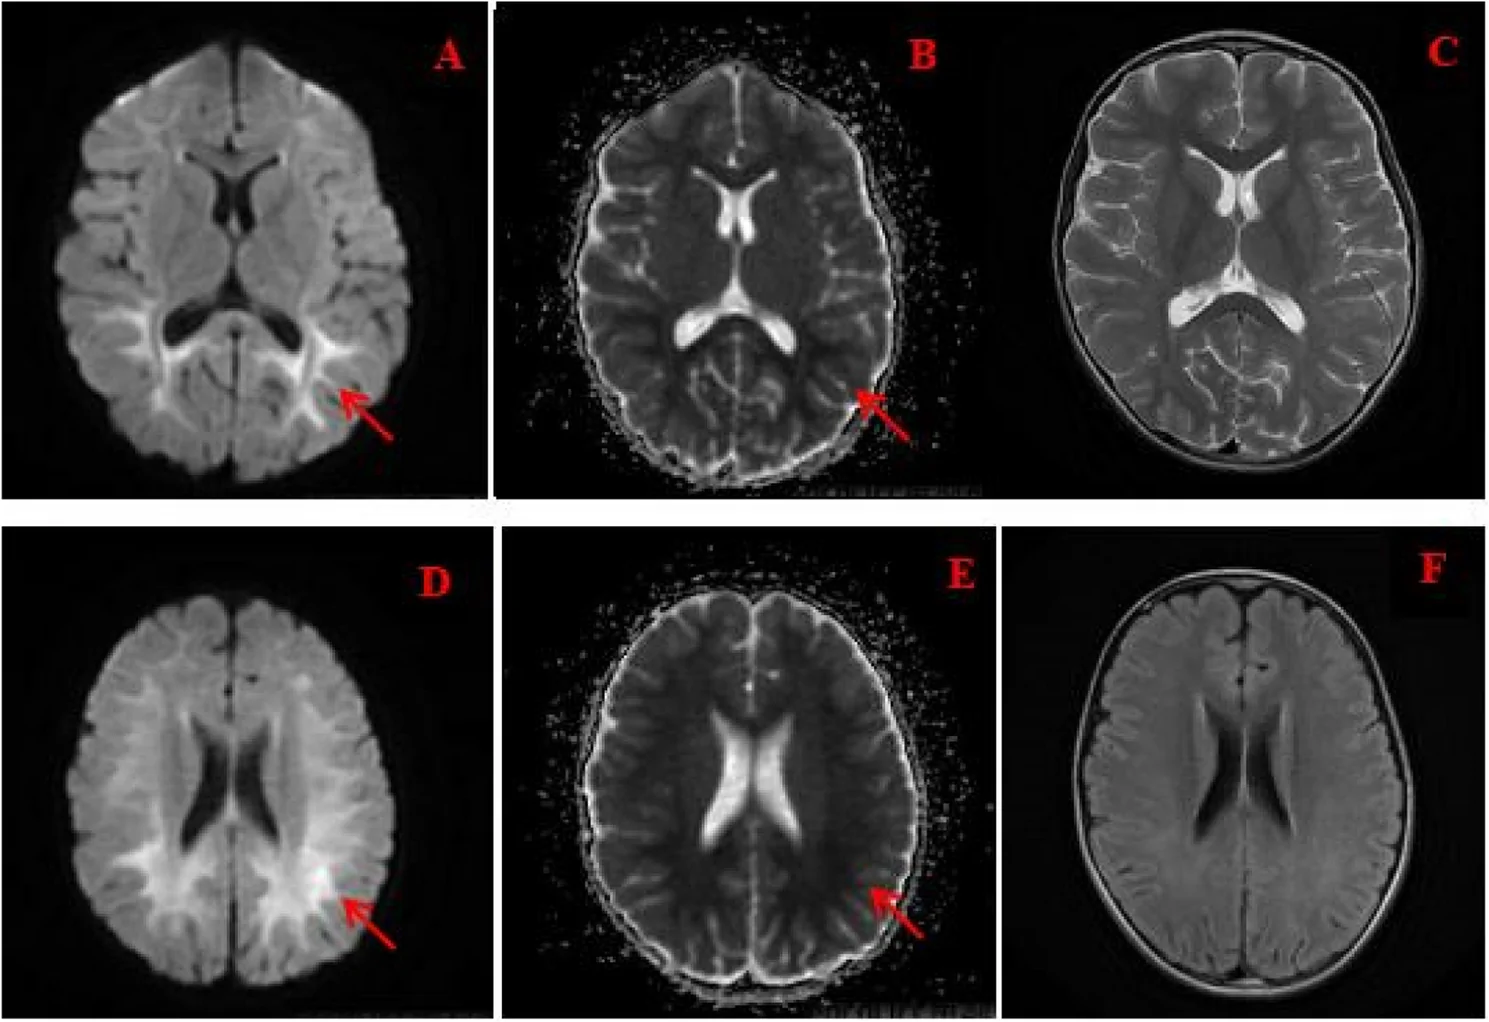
Brain MRI results of children with hypersarcosinemia(January 26, 2025, 14 days after admission and following clinical recovery). A and D: Large patches of DWI hyperintense signal in the subcortical white matter of the bilateral cerebral hemispheres were observed, and the range was larger than before (arrows). B and E: Large patches of subcortical white matter in the bilateral cerebral hemispheres showed hypointense on ADC maps (arrows). C: The T2-weighted image showed no abnormalities. F: The FLAIR image showed no abnormalities. MRI, magnetic resonance imaging. DWI, diffusion-weighted imaging. ADC, apparent diffusion coefficient. FLAIR, fluid-attenuated inversion recovery
